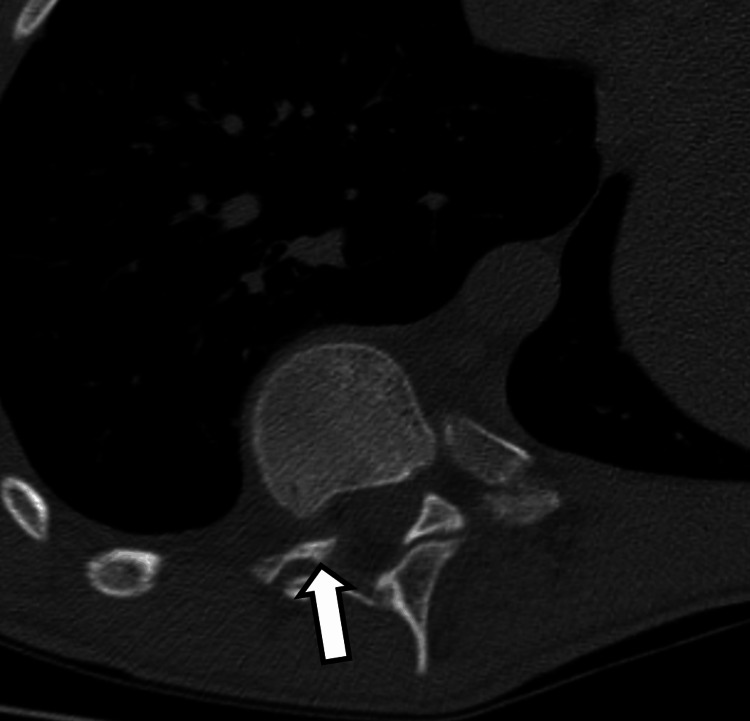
Axial CT image in bone kernel through T9-T10 neural foramen showing protrusion of the pointed head of the rib through the foramen into the spinal canal (arrow).
